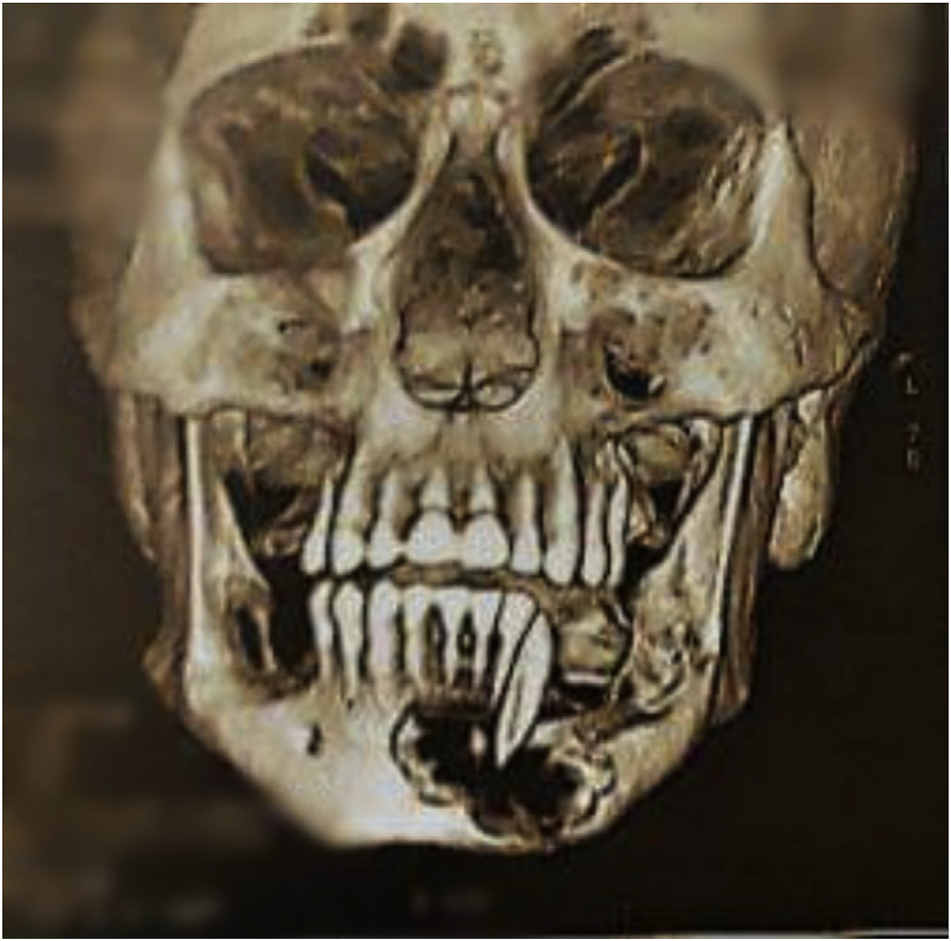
CT scan with 3D reconstruction: frontal incidence showing multiple radiolucent areas with well outlined borders on the mandible with a fracture and on the maxilla.
